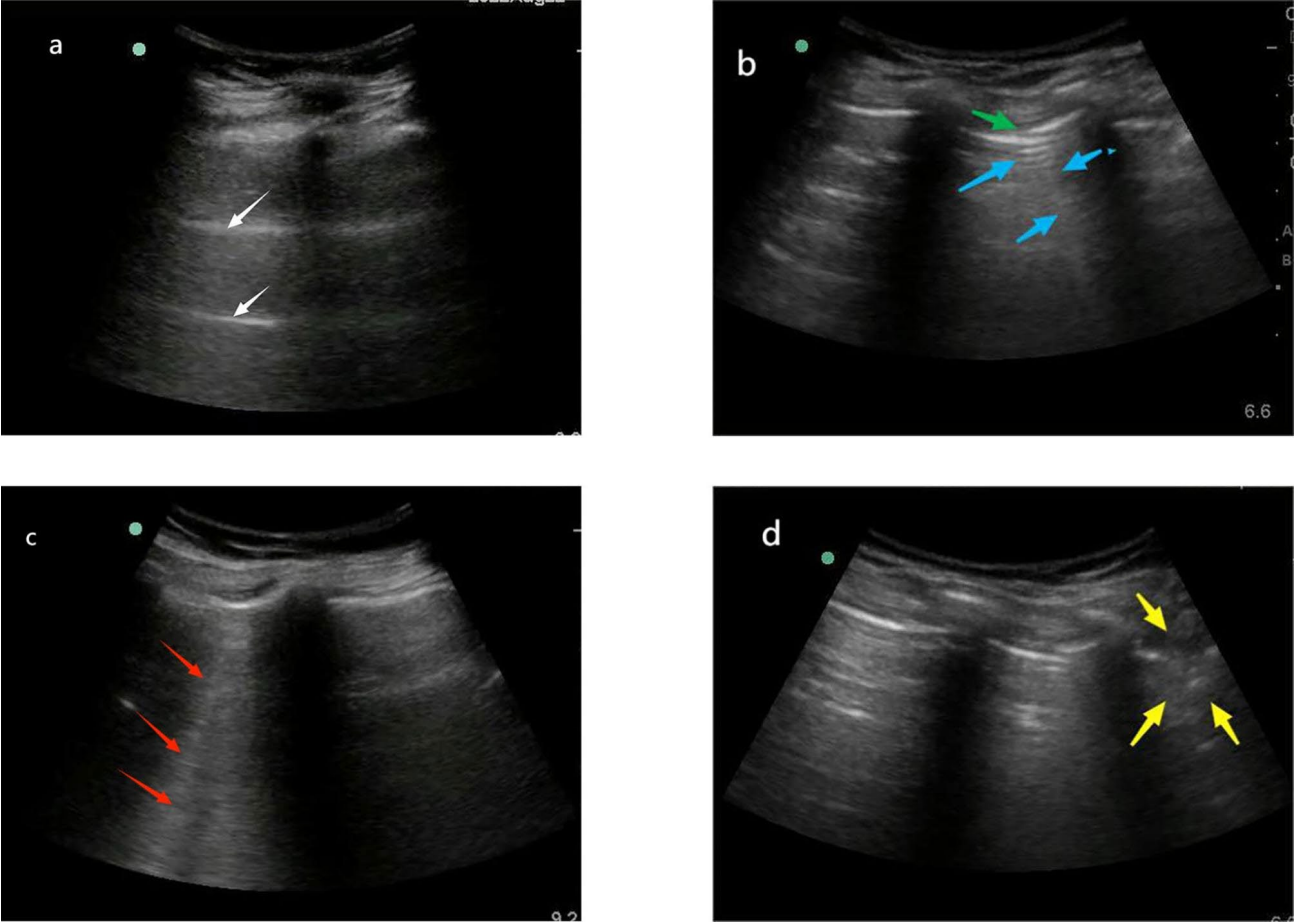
Lung ultrasound images with different scores. Note: The white arrow is the A line; the blue arrow is the B line; the red arrow is the gathering B line; yellow arrows are subpleural nodules; the green arrow is the discontinuous pleura. The above figure shows the ultrasound images of the patients with different scores. Figure A: normal pleura and A line, rated as 0; Figure B: Discontinuous pleura and multiple B lines, rated as 1 point; Figure C: Several gathered B lines, rated as 2 points; Figure D: Subpleural consolidation with diameter > 2 cm, rated as 3 points
